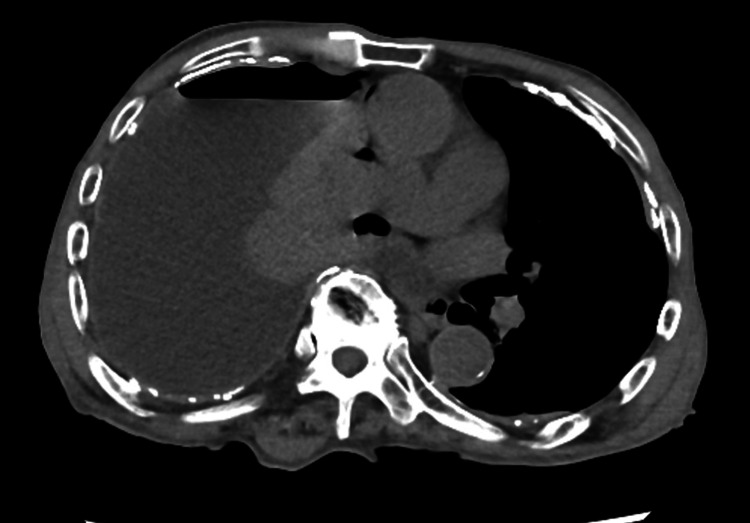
CT revealed a significant pleural effusion on the right side and multiple pleural plaques.
